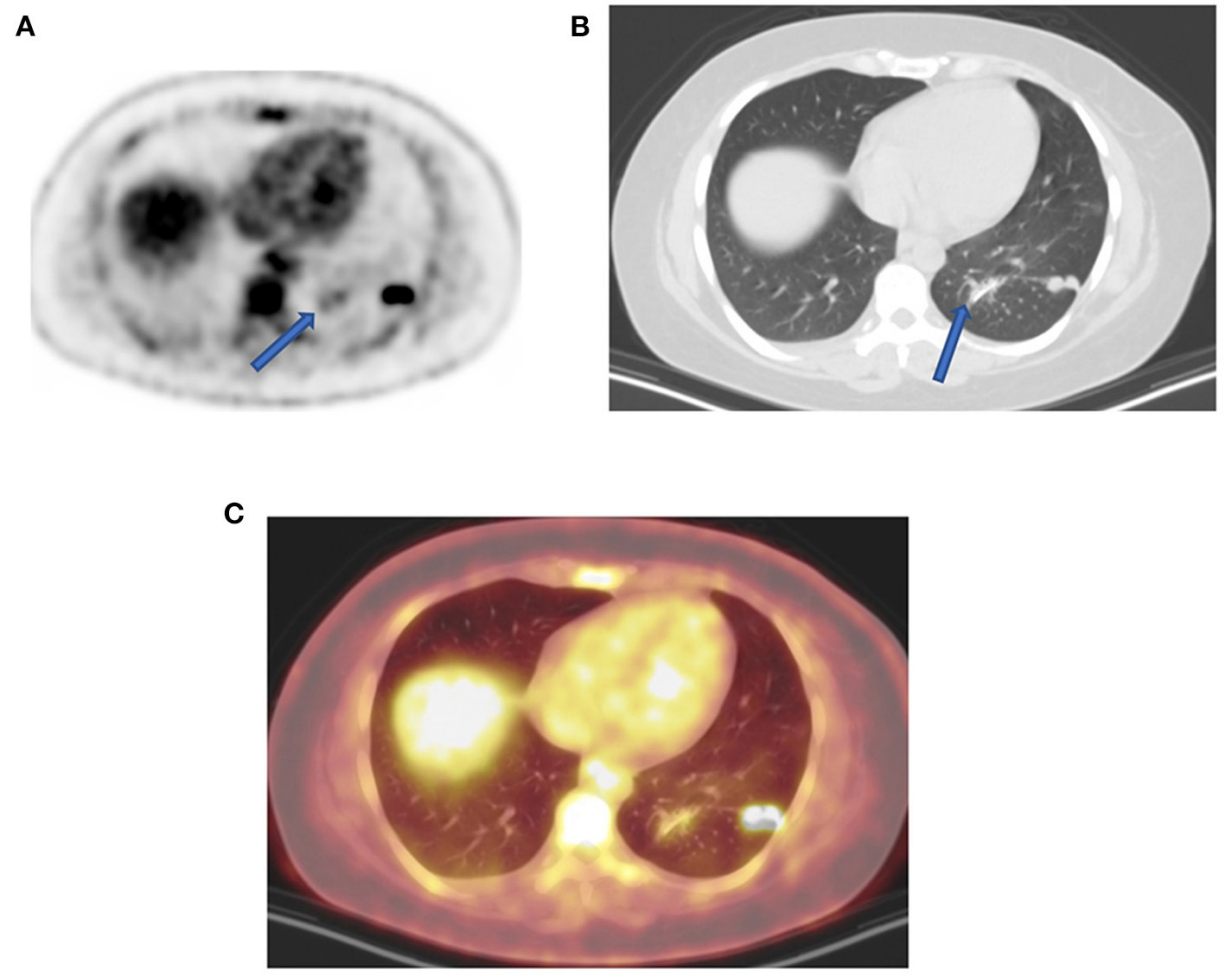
Uptake due to unusual attenuation correction. FDG PET axial (A) attenuation-corrected image shows intense FDG uptake in the posterior lateral aspect of the left lung and moderate uptake medially (arrow) caused by an artifact induced by attenuation correction. Corresponding lung window CT (B) shows sutures (arrow) from previous surgery (13 months earlier) as confirmed on combined PET-CT (C) corresponding plane. Without CT anatomy, the uptake caused by attenuation correction could mimic pulmonary pathology adjacent to real lung active nodules. In the presence of potential attenuating elements such metal implant, surgical sutures, and staples with unexplained FDG uptake, it is upon interpreting physicians to evaluate corresponding non-attenuation-corrected images to minimize reporting errors.
